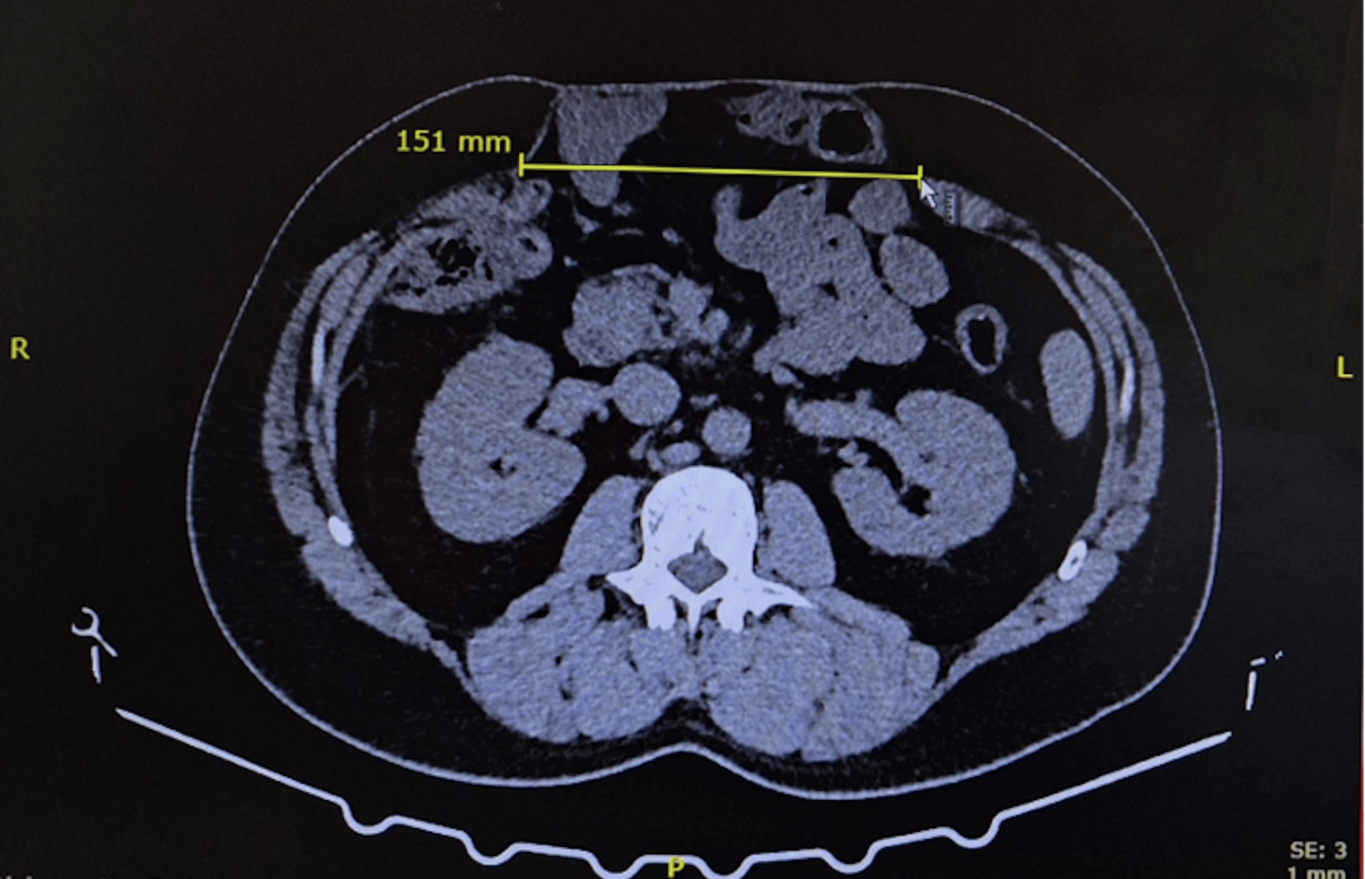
Abdominal CT scan of showing a 15.1 cm × 18.2 cm aponeurotic defect. The image shows the hernial orifice with a maximum width of 15.1 cm in the mesogastric region. A hernial sac containing loops of the small intestine and adipose tissue is also identified.
